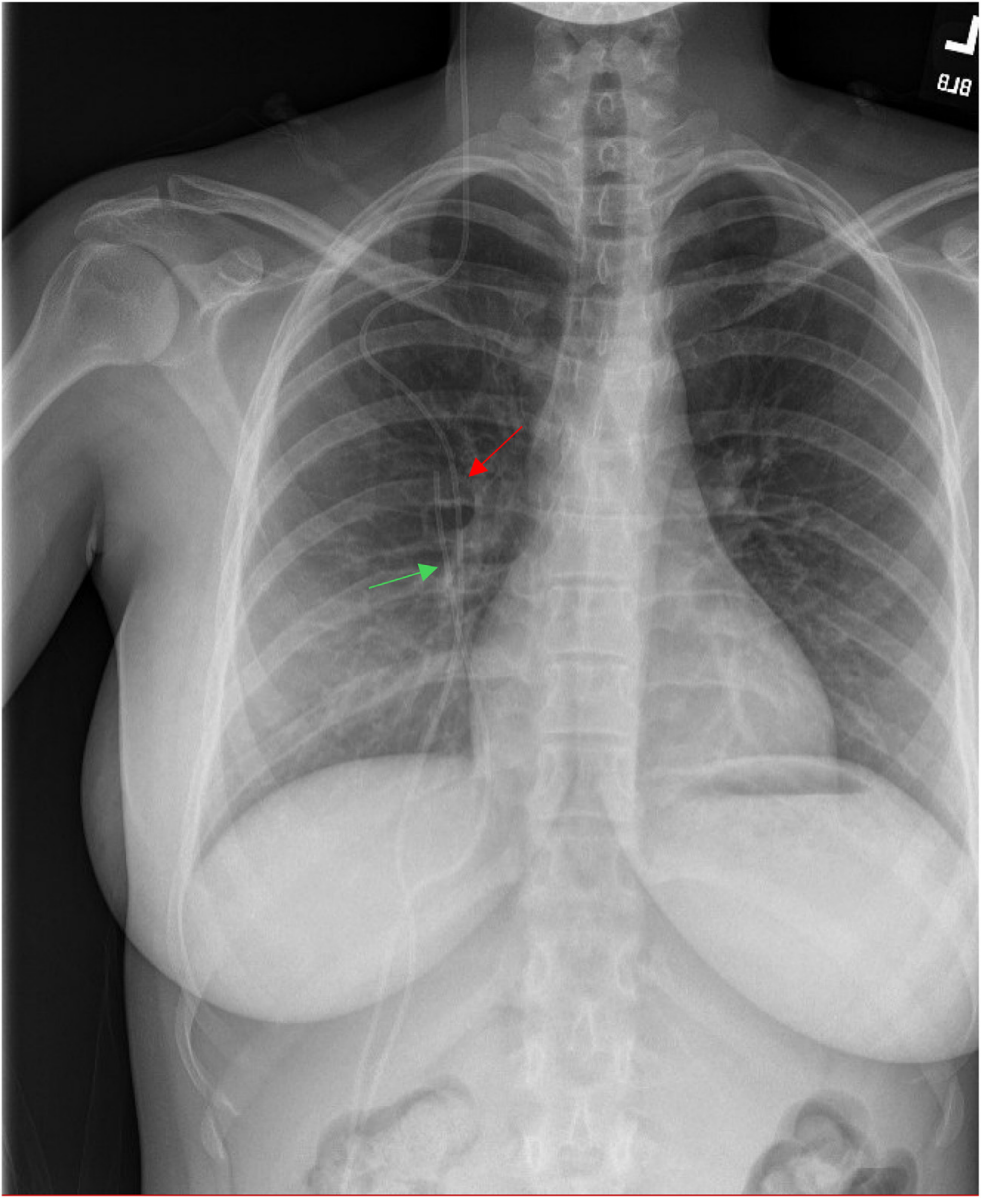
Postoperative X-ray showing the previous (green) and current (red) VP shunt positions.
